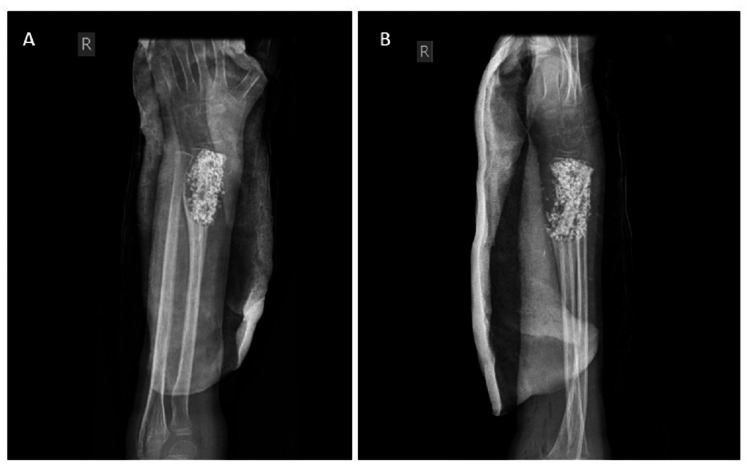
Immediate postoperative check X-ray: (A) AP view showing cavity filled with fibular strut graft and synthetic bone graft and the articular surface is intact and (B) lateral view showing the grafts without any articular surface breach
